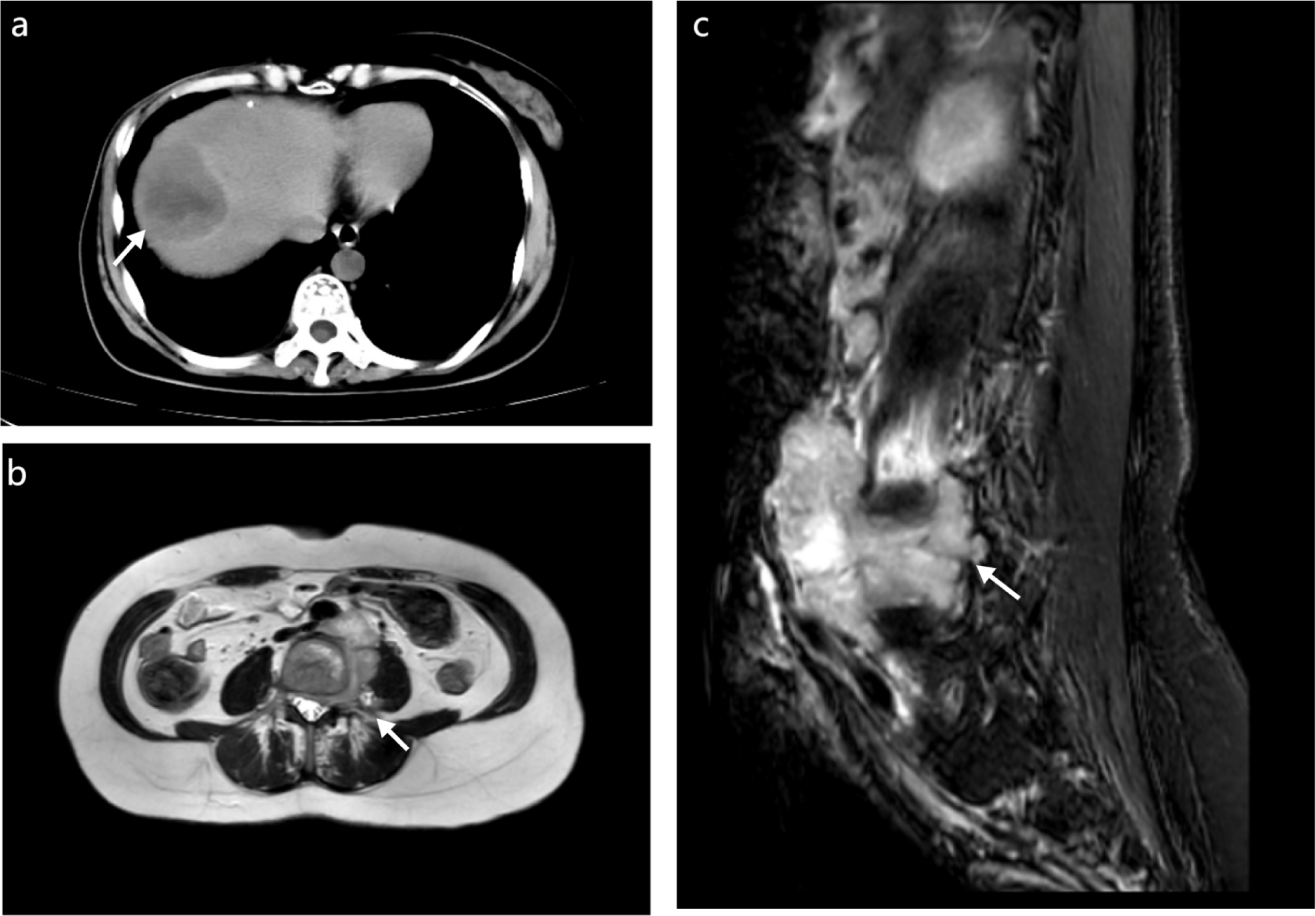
Non-contrast computed tomography of the upper abdomen shows a hypodense lesion in the right hepatic lobe, consistent with hepatic metastasis (a). Axial magnetic resonance imaging demonstrates a pelvic mass involving the adnexal region and vertebral metastasis (b). Sagittal T2-weighted MRI of the lumbar spine reveals an osteolytic lesion involving the vertebral body, indicating bone metastasis (c).
